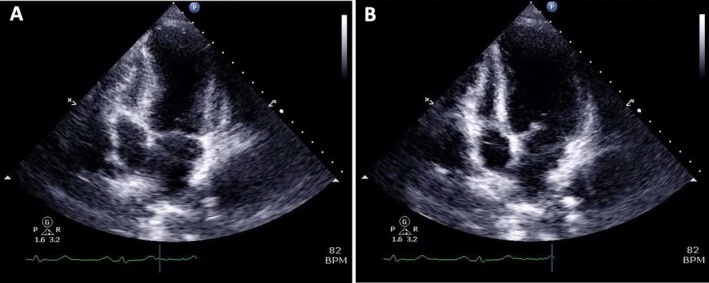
Transthoracic echocardiography (TTE), A4C view during (A) systole and (B) diastole. They revealed mid anterolateral, inferolateral, and all apical segments hypokinesia with preserved basal wall motion, consistent with the diagnosis of TC.
